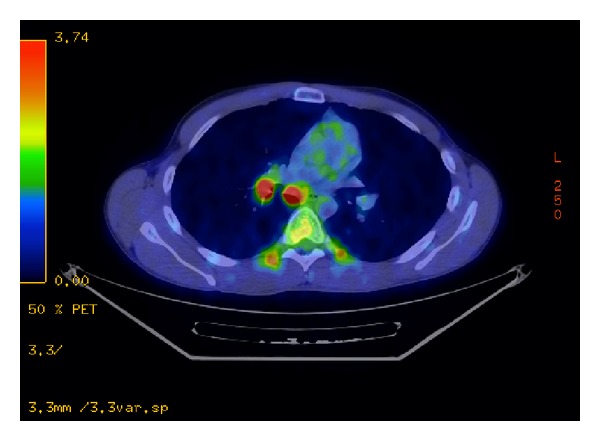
PET CT demonstrates hypermetabolic mediastinal lymph nodes.
